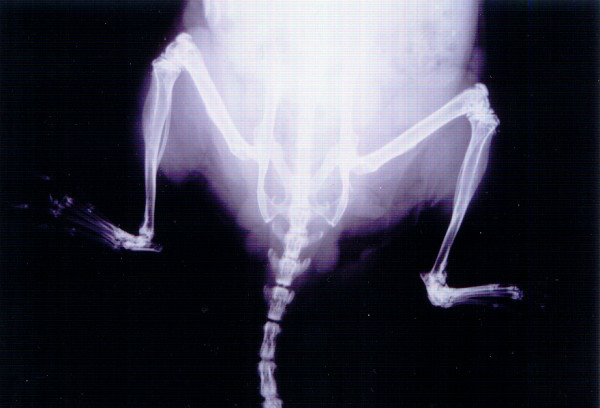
A radiograph of ANKENT-positive male. A control LPS-untreated male with the right paw affected. The left paw is normal.
